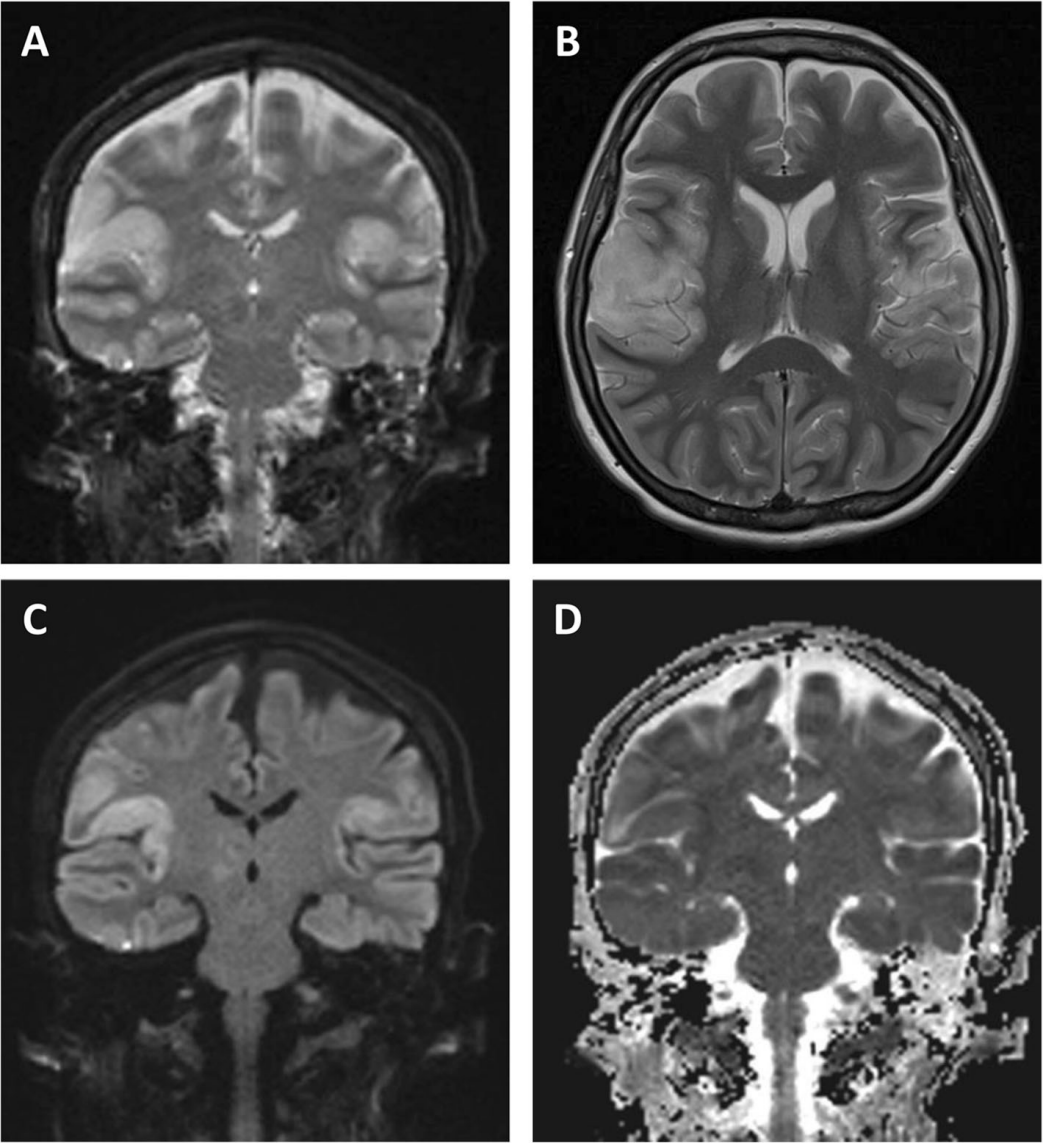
cMRI imaging. Cortical/subcortical oedema within insular and parietal lobe on coronal and axial T2w (a, b), coronal DWI with increased signal on B1000 image (c) and decreased ADC (apparent diffusion coefficient) (d) within the encephalitic lesion
